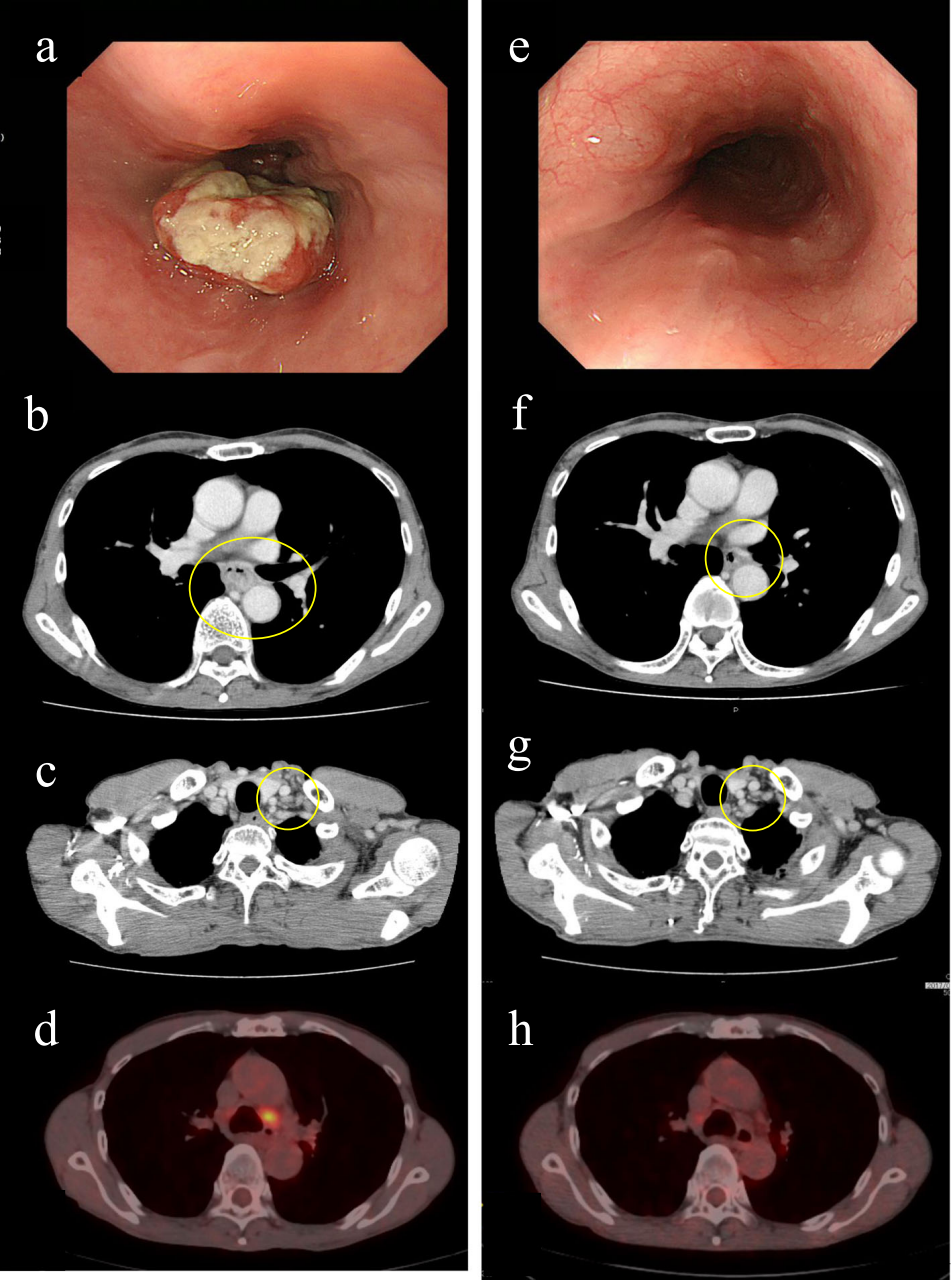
Endoscopy and imaging before neoadjuvant chemotherapy (NAC) for esophageal cancer (a–d) and the findings after NAC (e–h). Endoscopy revealed marked shrinkage of the primary tumor (a, e). Computed tomography also revealed contraction of the primary tumor (indicated by a yellow circle in b, f). The sizes of the cervical lymph nodes defined as no. 104 by the Japanese Esophageal Society were stable after NAC (indicated by a yellow circle in c, g). Positron emission tomography also decreased uptake in the primary tumor (d, h)
